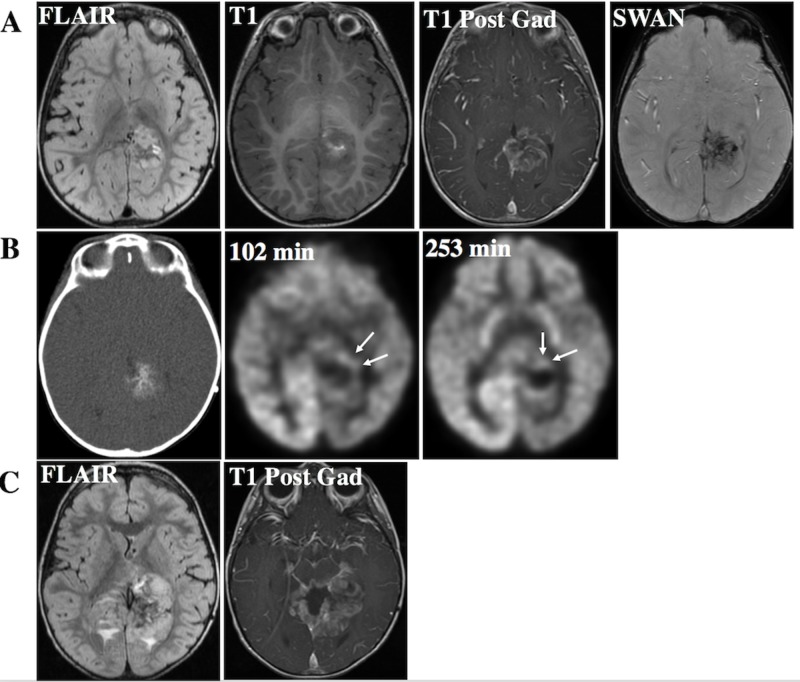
Pineoblastoma with the DICER mutation on follow-up A. FLAIR hyperintense and enhancing lesion within the left posterior cingulate gyrus with the involvement of the left hippocampus and splenium of the corpus callosum. The lesion demonstrates an intrinsic T1 hyperintense signal on T1-weighted imaging and a susceptibility artifact on Swan, indicating the presence of blood products. B. PET CT with FDG tracer uptake acquired 102 and 253 minutes after the injection demonstrates prominent focal uptake surrounding the region of the calcified tumor. C. Follow-up imaging confirms disease progression with interval increase in the size of the tumor and the surrounding enhancement. FLAIR: fluid attenuated inversion recovery; FDG: fludeoxyglucose; PET: positron emission tomography
